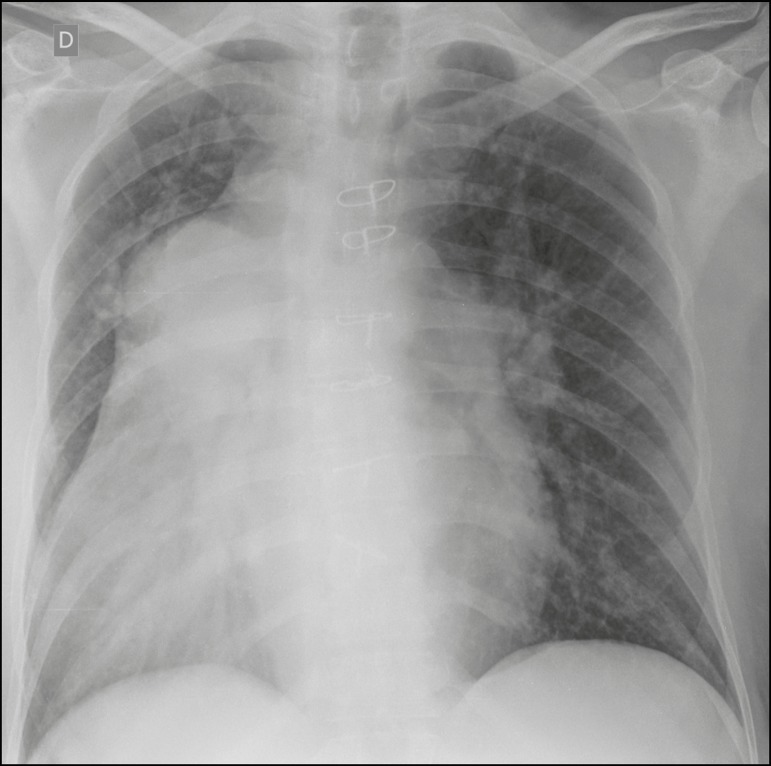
Chest X-ray showing dextrocardia, a widened mediastinum, and enlarged
